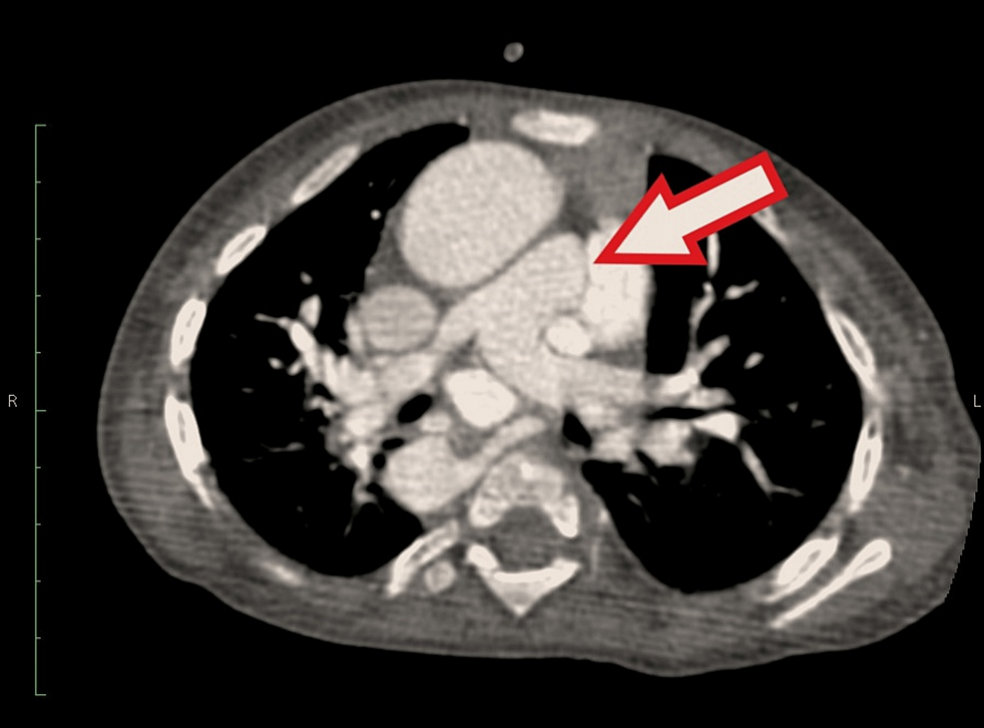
Computed tomography pulmonary angiogram revealing main pulmonary artery and pulmonary valve stenosis (arrow).
